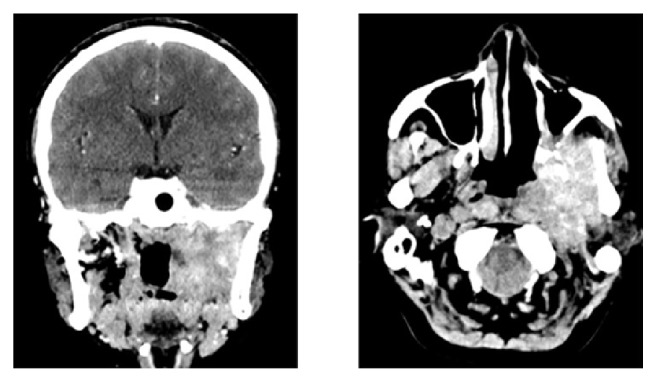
A 27-year-old woman complaining of head pain and trismus was diagnosed with IMT of the parapharyngeal space. Several biopsies were conducted before the diagnosis was confirmed. The contrast-enhanced CT showed the intense enhancement of the tumor surrounding the important vessels. She did not opt for the treatment after being informed of the benefits and risks of different treatments. After 37-month follow-up, no symptomatic progression was seen.
